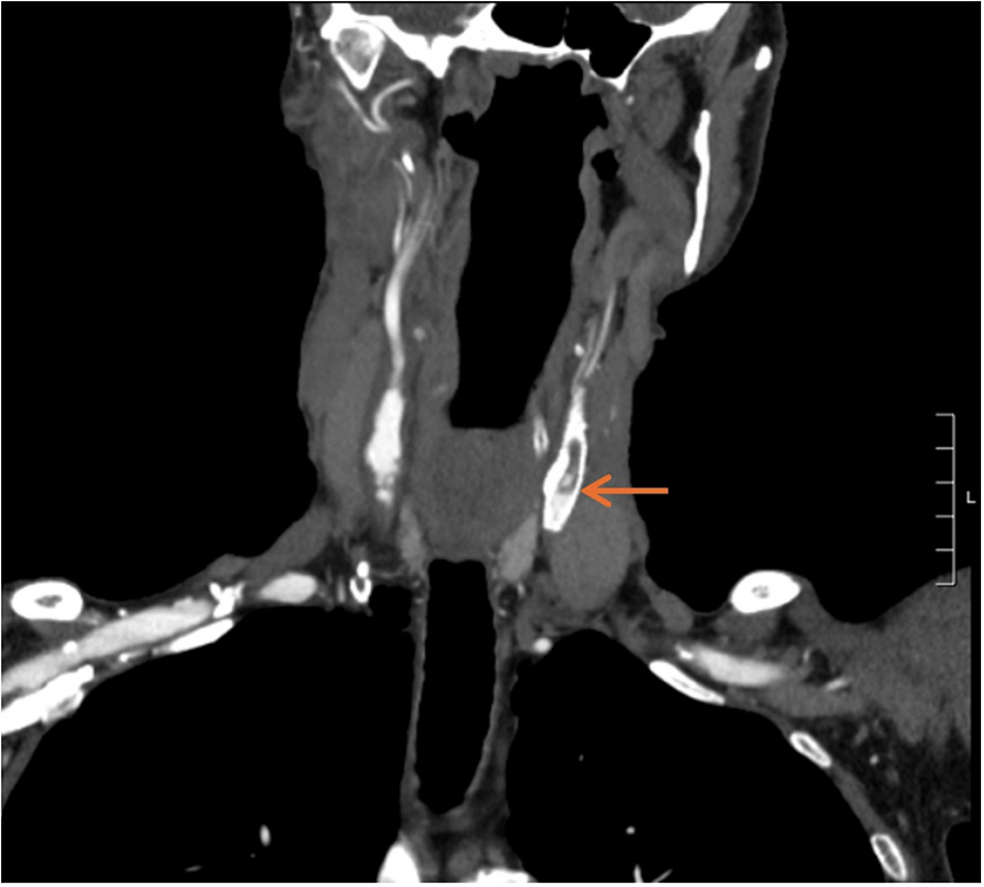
Coronal view of a CT angiogram depicting in-stent restenosis of left common carotid artery stent (arrow).
